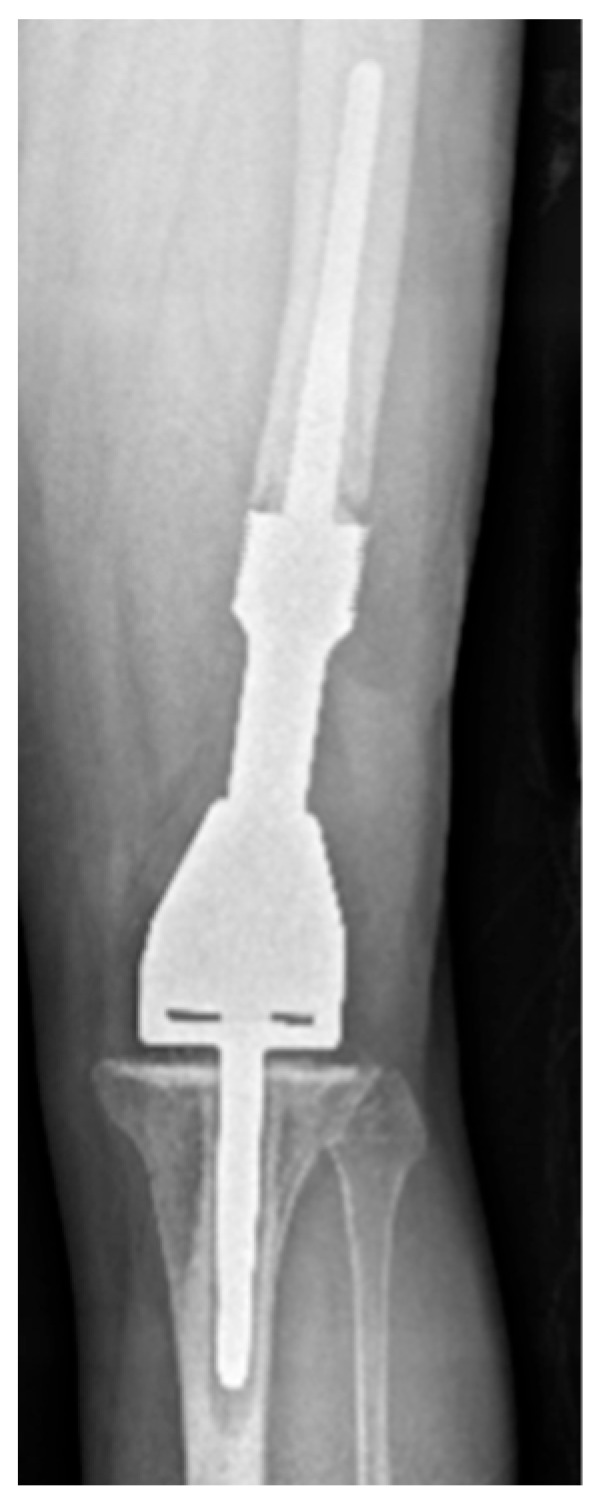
AP radiograph showing grade 2 loosening of EPR, loosening at the shoulder of femoral component.
